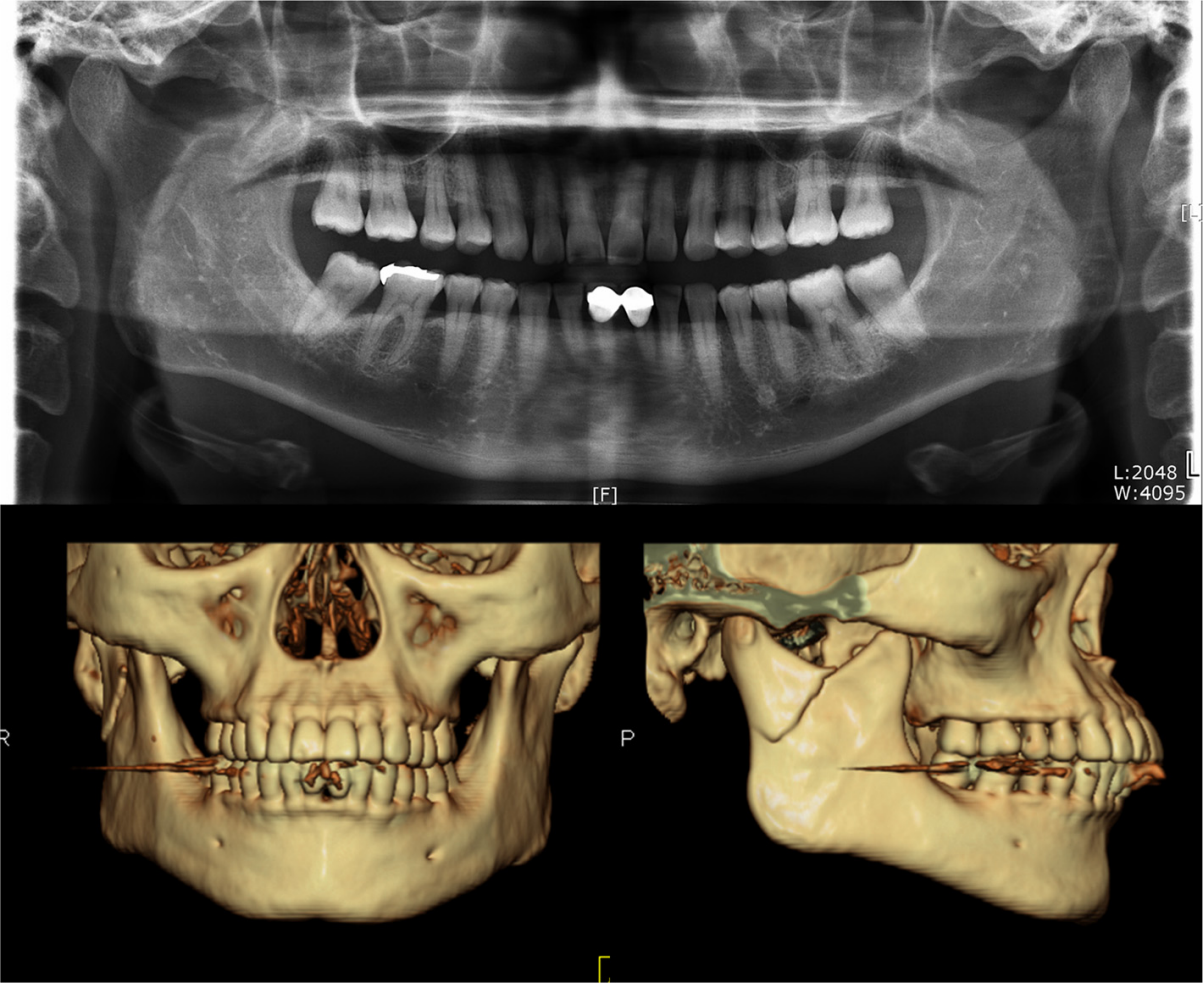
Preoperative: panoramic radiograph (*above*) and 3D-CT, frontal and right lateral view, (*below*) showing the fractured right subcondyle and prominent angle of mandible
